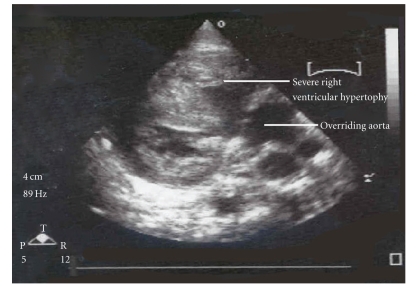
Echocardiogram of Twin B showing overriding aorta and right ventricular hypertrophy.
